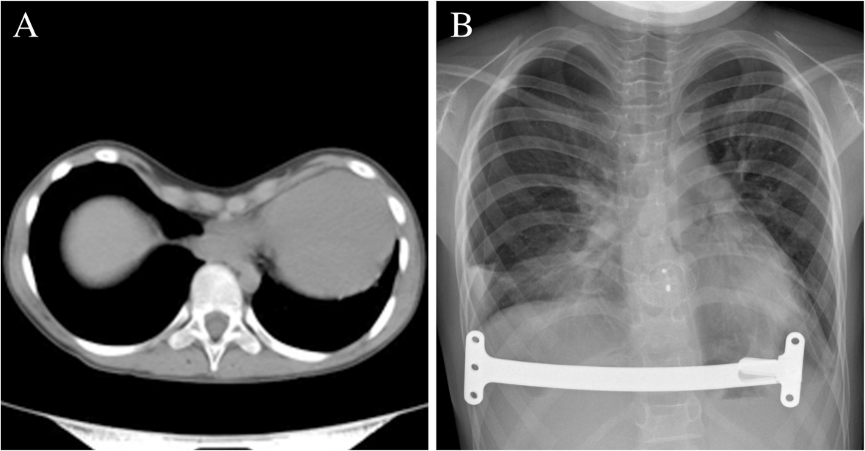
Imaging of the pectus excavatum. (A) CT showing the severe asymmetric pectus excavatum with severe cardiac compression and displacement. (B) X-ray showing the strutting bar that corrected the funnel chest.
